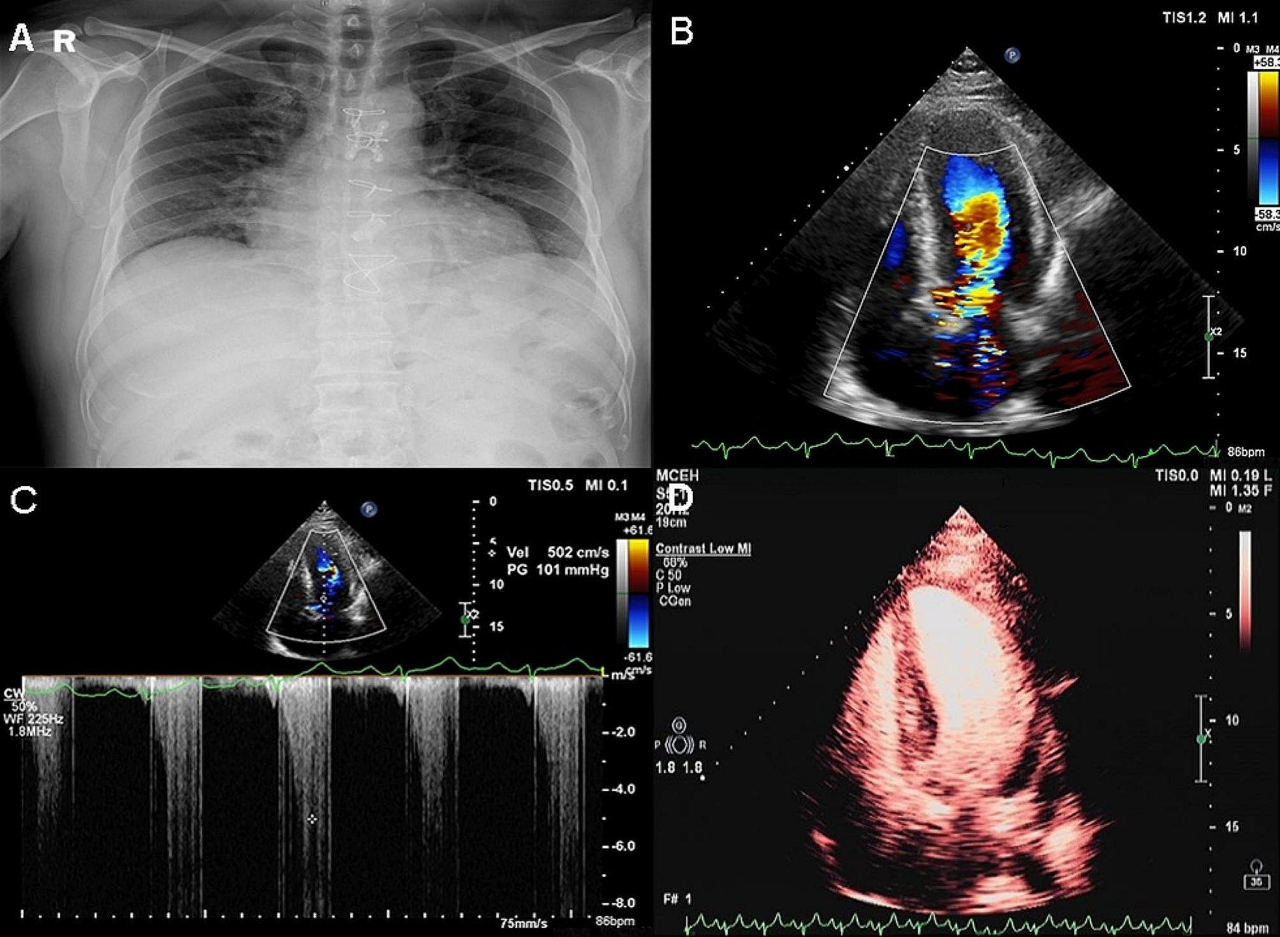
A: Chest X-ray; B: Color Doppler flow.; C: The systolic pressure gradient of the LVOT. D: Left ventricular acoustic contrast
